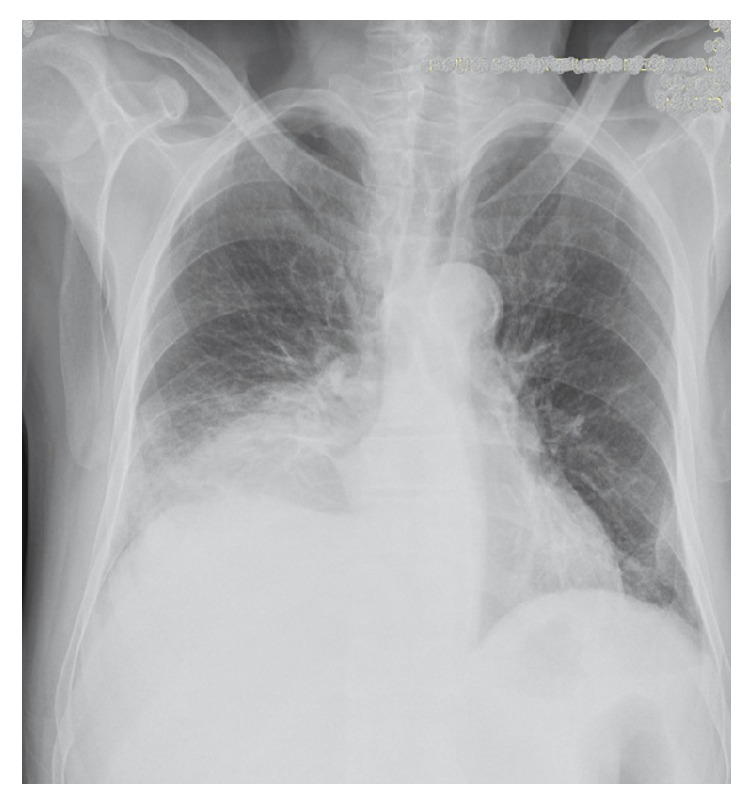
Pneumonic infiltration on chest X-ray.
